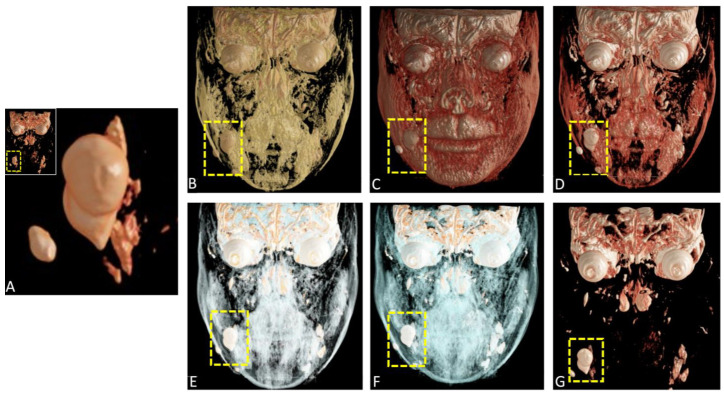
Preoperative coronal cinematic rendering (CR) reconstructions from magnetic resonance imaging (MRI) datasets using a variety of presets (A–G). The integration of various presets further optimizes the representation of superficial structures, contributing to improved diagnostic accuracy and aiding in surgical planning.
